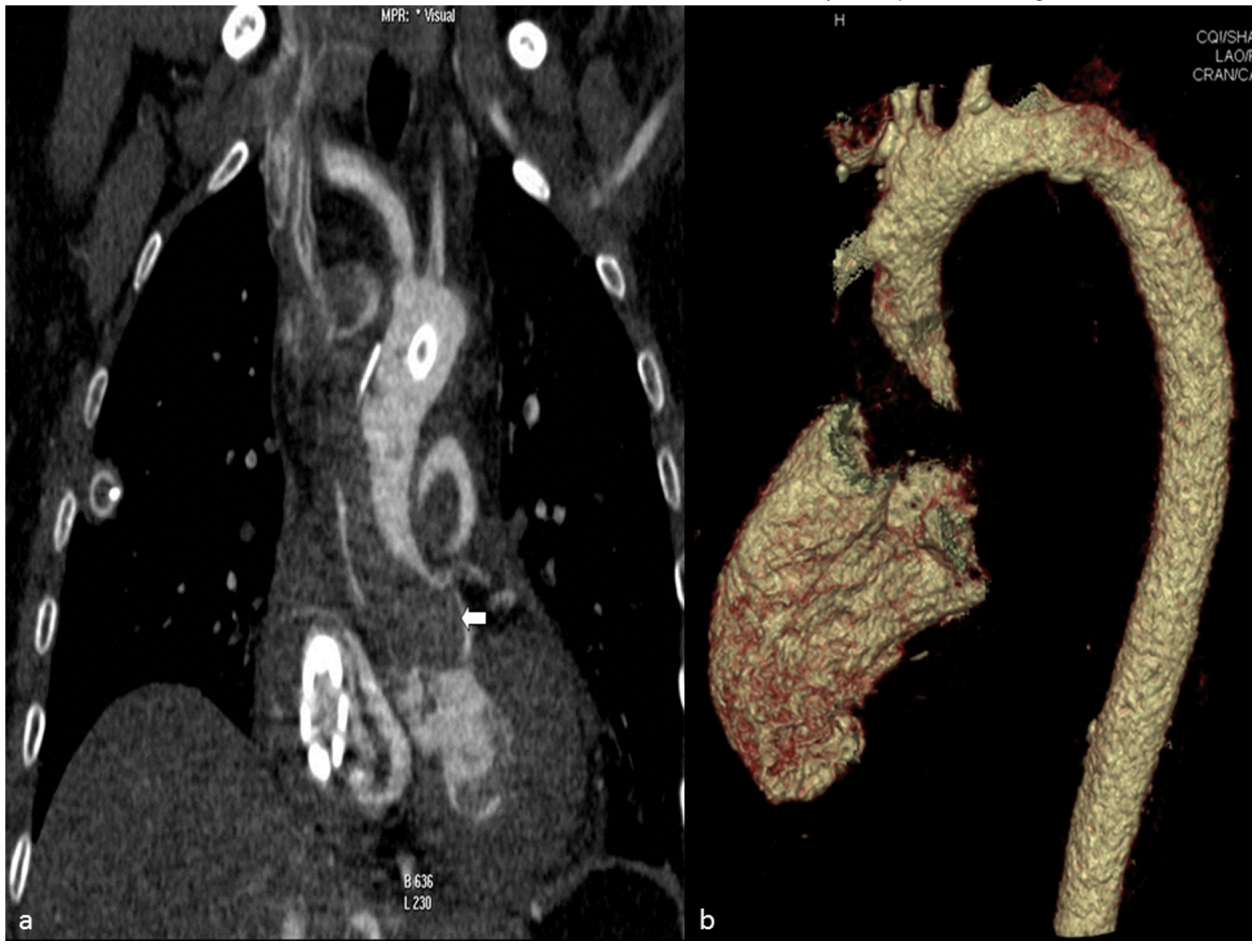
a: paracoronal view of the chest, soft tissue-windowing. Thrombosis of the ascending aorta with occlusion of coronary arteries can be seen (white arrow). Conventional autopsy later confirmed the diagnosis. b: 3D reconstruction of CT of the same patient shows lack of contrast between left ventricle and ascending aorta due to thrombosis.
